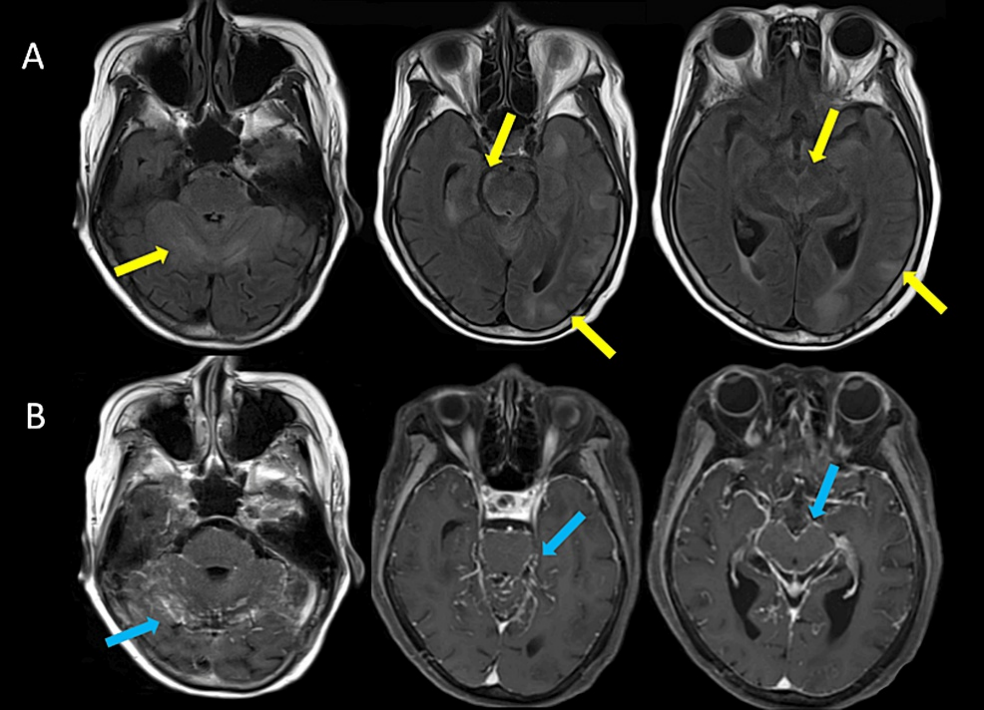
Brain MRI on admission A: FLAIR images showing showed multiple T2 high-intensity lesions on the surface of the cerebellum, brainstem, and cortex (yellow arrows); B: T1-weighted images with gadolinium enhancement (T1Gd) in which pia-arachnoid membrane and vessel walls of the brain surface showed a marked gadolinium-contrast enhancement effect (blue arrows). FLAIR: fluid-attenuated inversion recovery
